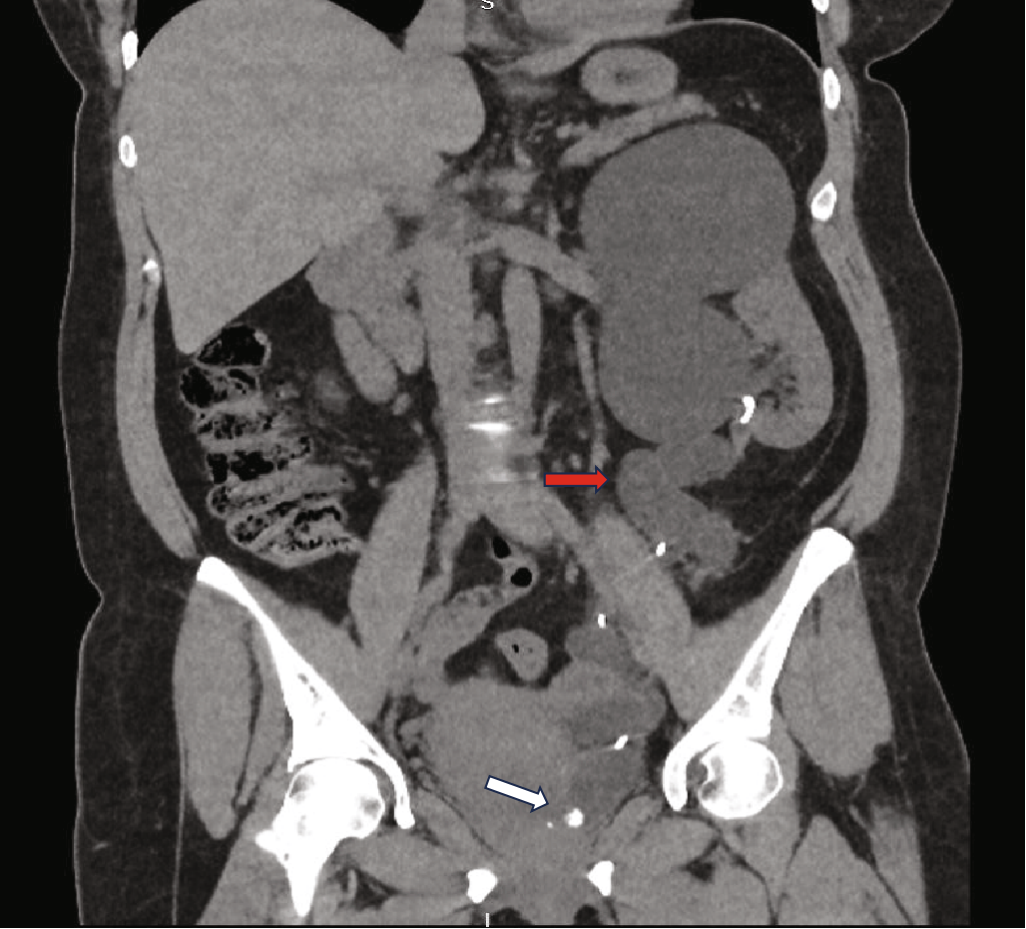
Preoperative CT-KUB: coronal section. Dilated tortuous ureter from upper hydronephrotic moiety (red arrow). Distal calculus on upper moiety dilated ureter (no clear point of upper moiety ureteric orifice on the CT scan—white arrow).
